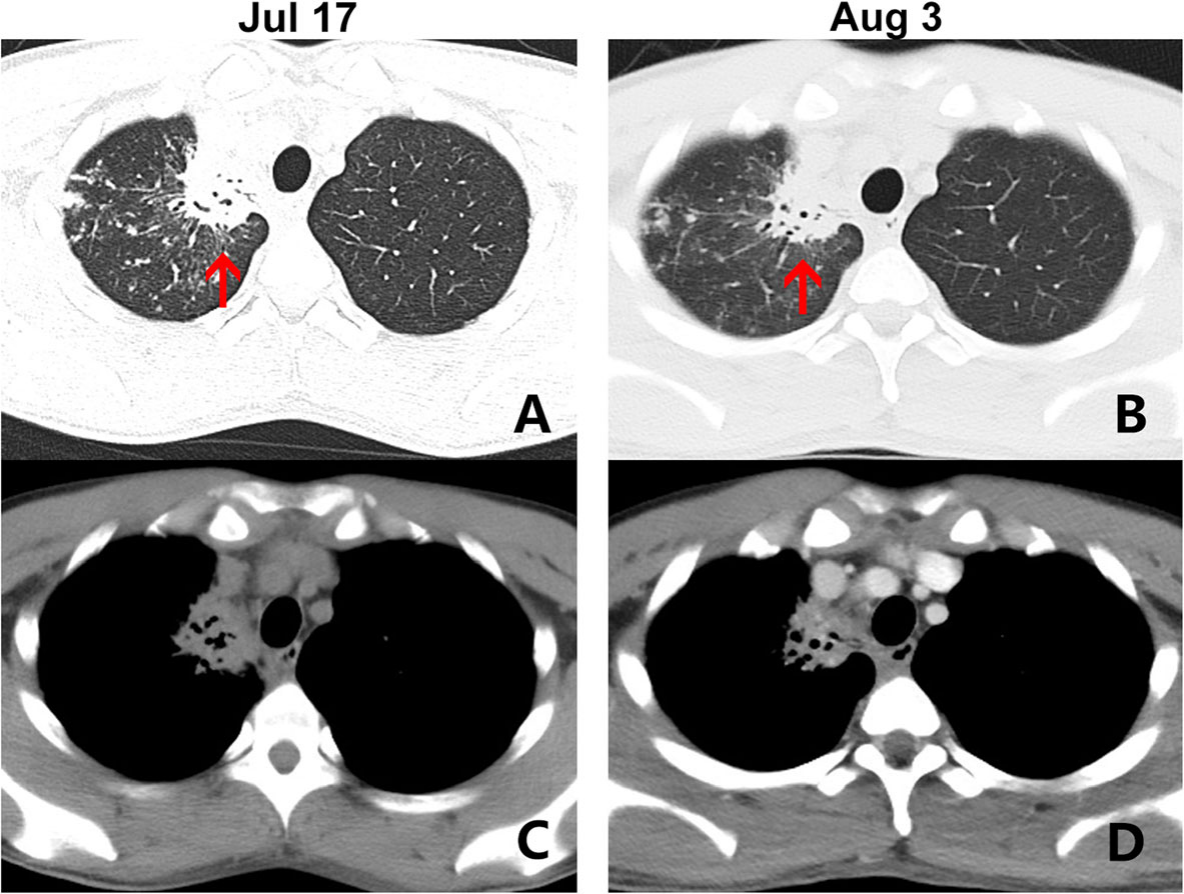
Chest CT scan of the lungs before admission. a A 30 × 37 mm-sized mass-like inflammatory lesion (red arrow) with air space located at the right upper lobe on lung window on July 17. c The same section on diaphragm window. b The same mass-like lesion (red arrow) with 32 × 28 cm size, irregular boarder and marginal long spiculation located at the right upper lobe on lung window on August 3. d Same section on diaphragm window
